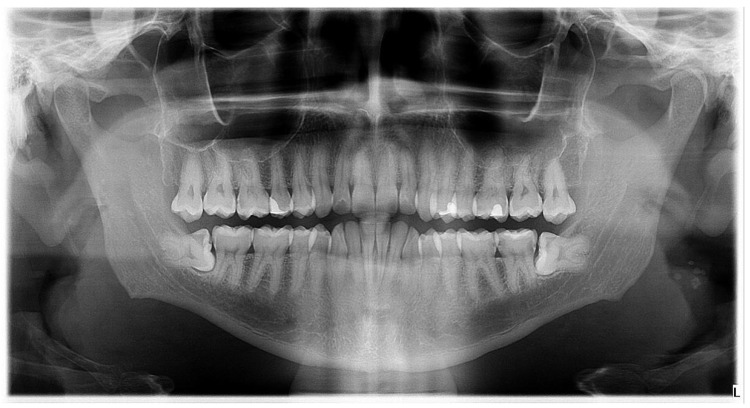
A panoramic X-ray taken before the alveolectomy of the lower right third molar.
